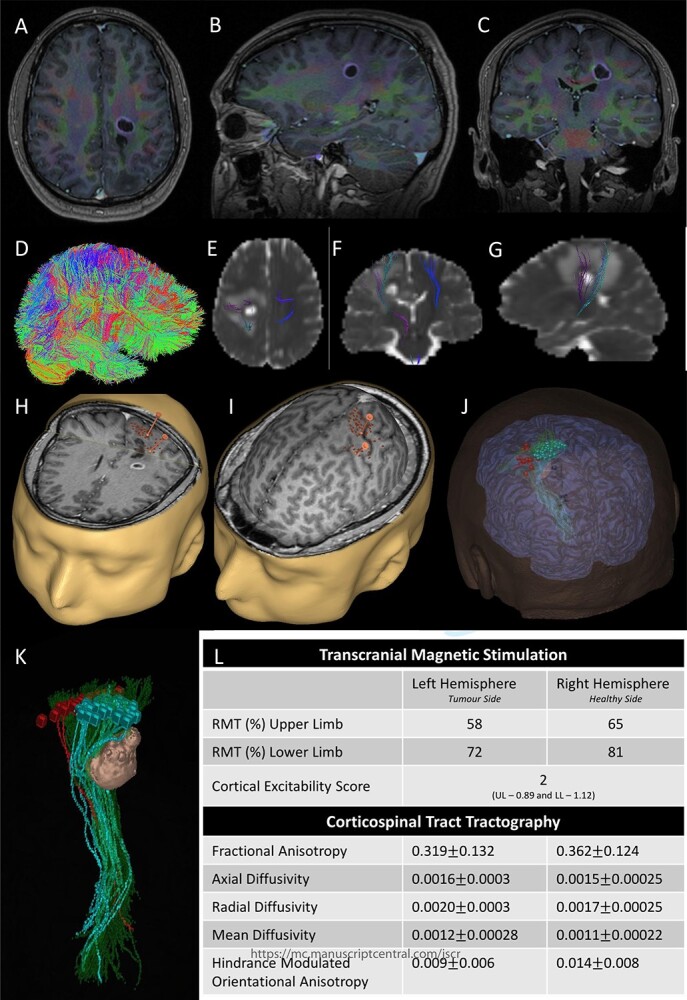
Integrated preoperative anatomical and functional brain mapping; (A–C) axial, sagittal and coronal fusion of T1-weighted MRI images with gadolinium and first eigenvector fractional anisotropy (FEFA); (D) whole brain tractography performed with StarTrack and visualized in TracViz according to deterministic spherical deconvolution algorithm. (E–G) fusion of CST with the ADC map; (H and I) nTMS mapping of the upper and lower limbs with hotspots for both upper and lower limbs identified with a marker (orange); (J and K) 3D Modelling of the tumour and the cortical and subcortical mapping of the CST with Stealth S8; (L) table summarizing the nTMS variables (resting motor threshold—RMT—and Cortical Excitability Score—Number of abnormal interhemispheric RMT ratios) and the tractography metrics.
